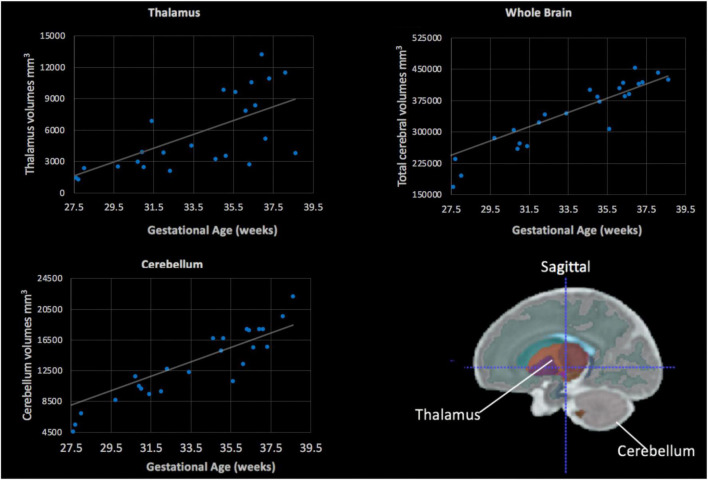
Fetal subcortical volumes (*y*-axis) plotted in relation to gestational age (*x*-axis). Fetal thalamus (top, left), whole brain (top, right) and cerebellum (bottom, left) volumes were plotted in relation to gestational age in weeks. The cerebellum, thalamus and total cerebral volumes showed a positive linear association with gestational age (all, *p* < 0.05). (Bottom, right) The color-coded fetal atlas overlaid on a atlas MRI demonstrates the location of the thalamus (orange/purple) and the cerebellum (gray).
